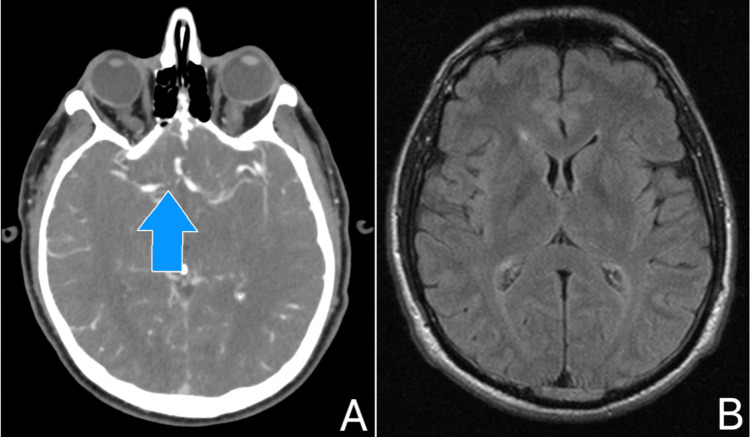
(A) Computed tomography angiography of the brain demonstrating a hypoplastic right basilar artery (blue arrow). (B) MRI scan of the brain with intravenous contrast was insignificant.
